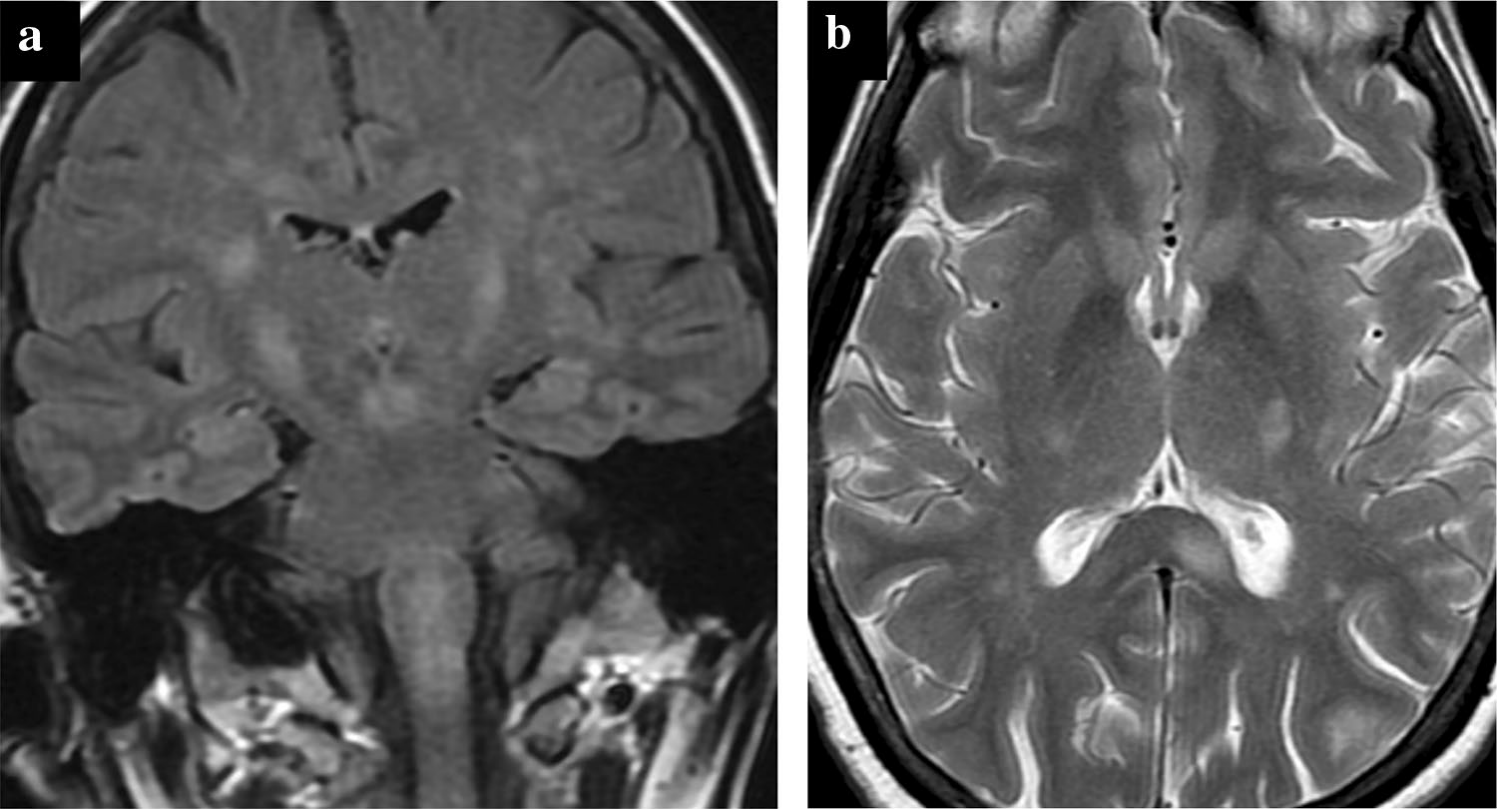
a, b Patient B MRI brain imaging during second episode of encephalitis. a Coronal FLAIR and b axial T2 FSE. New patchy signal change in the fronto-parietal white matter, splenium of the corpus callosum, cortico-spinal tracts and recrudescence of the diffuse brain stem signal change seen during the previous encephalitic episode
